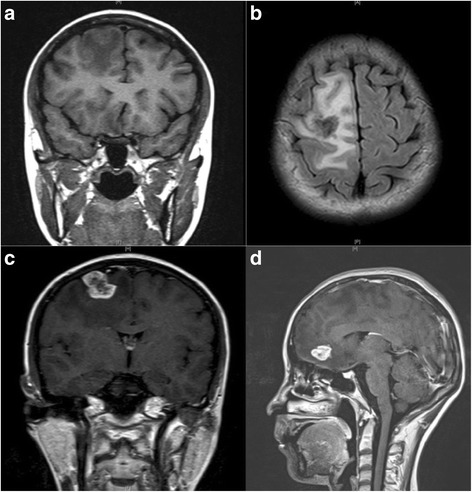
MRI scan of brain: a Coronal view of T1 W flair. The lesion was with low signal intensity on T1 W flair image. b Axial view of T2 W flair. The lesion was with low signal intensity and edema zone was seen around it on T2 W flair image. c Coronal view of T1 W flair + C. The lesion was with minimal rim enhancement. d Sagittal view of T1 W flair + C. The lesion was with minimal rim enhancement
